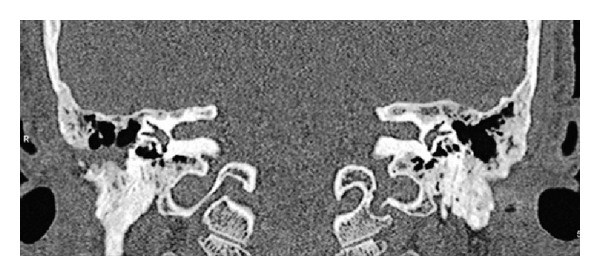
Postoperative CT scan. Note the absence of lytic lesion in left petrous apex and superior semicircular canal.
